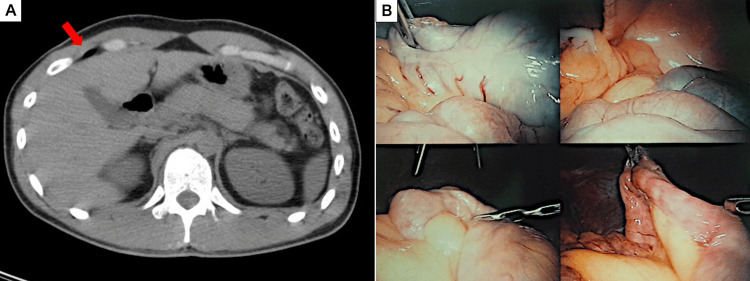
Imaging and intraoperative findings of Case 2. (A) Axial CT image revealing free air on the ventral side of the liver (red arrows). (B) A systematic laparoscopic survey of all abdominal quadrants confirming the absence of visceral injury or hemorrhage.
